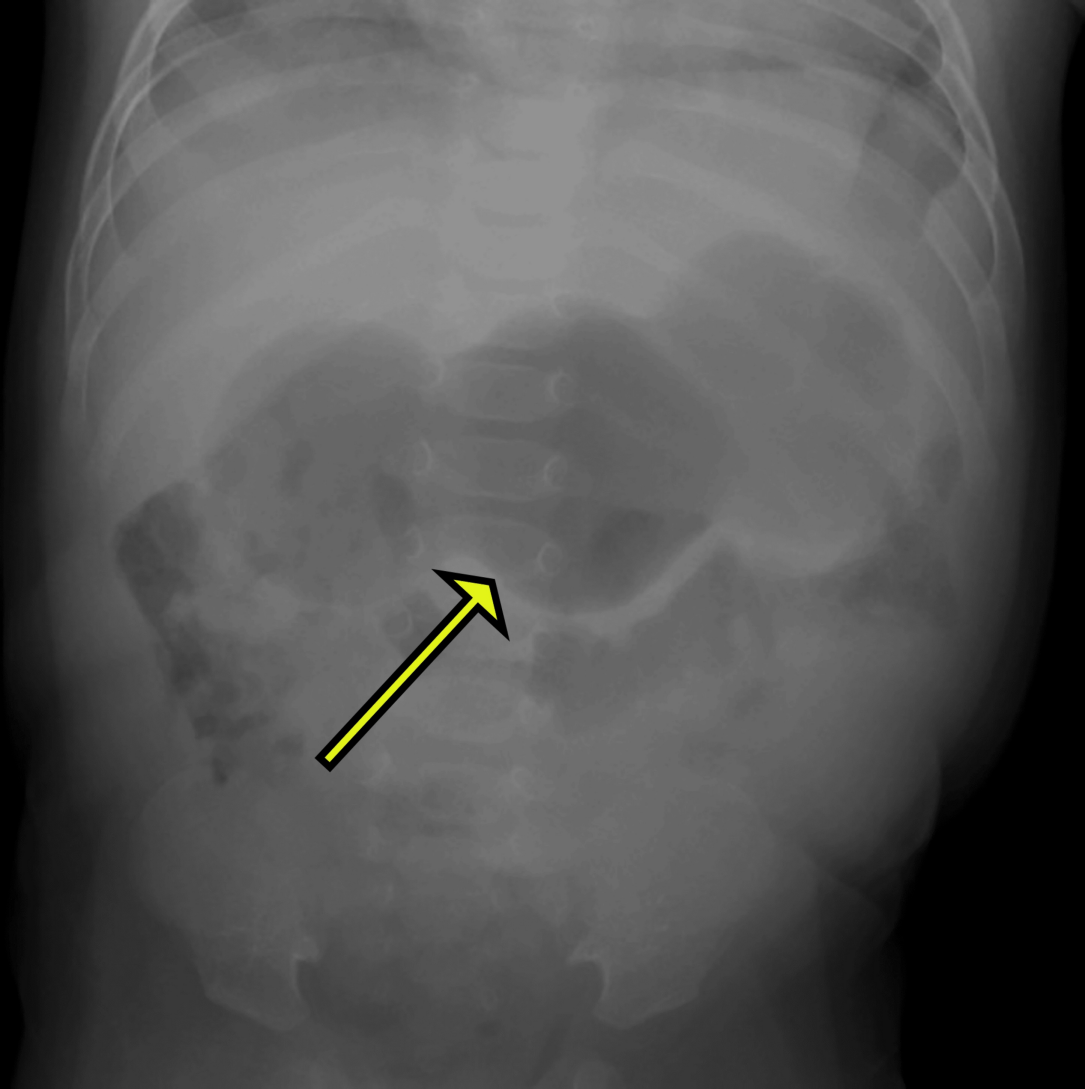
AP view radiograph of the abdomen shows a peristaltic dilated stomach giving the classic caterpillar sign (yellow arrow). AP, Anterior-Posterior view
